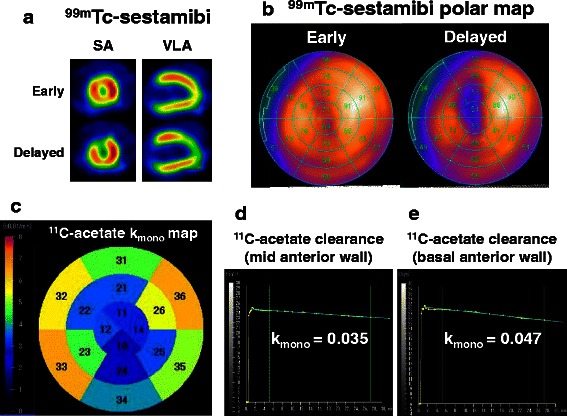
Representative case of an 85-year-old man who underwent emergent PCI for LAD. ^99m^Tc-sestamibi (MIBI) scintigraphy and ^11^C-acetate PET were performed 10 days after PCI. a Early and delayed images of MIBI SPECT. Accelerated MIBI clearance is observed in the anterior region. b Polar maps of MIBI in early (*upper*) and delayed (*lower*) images. c Oxidative metabolism (*k*
